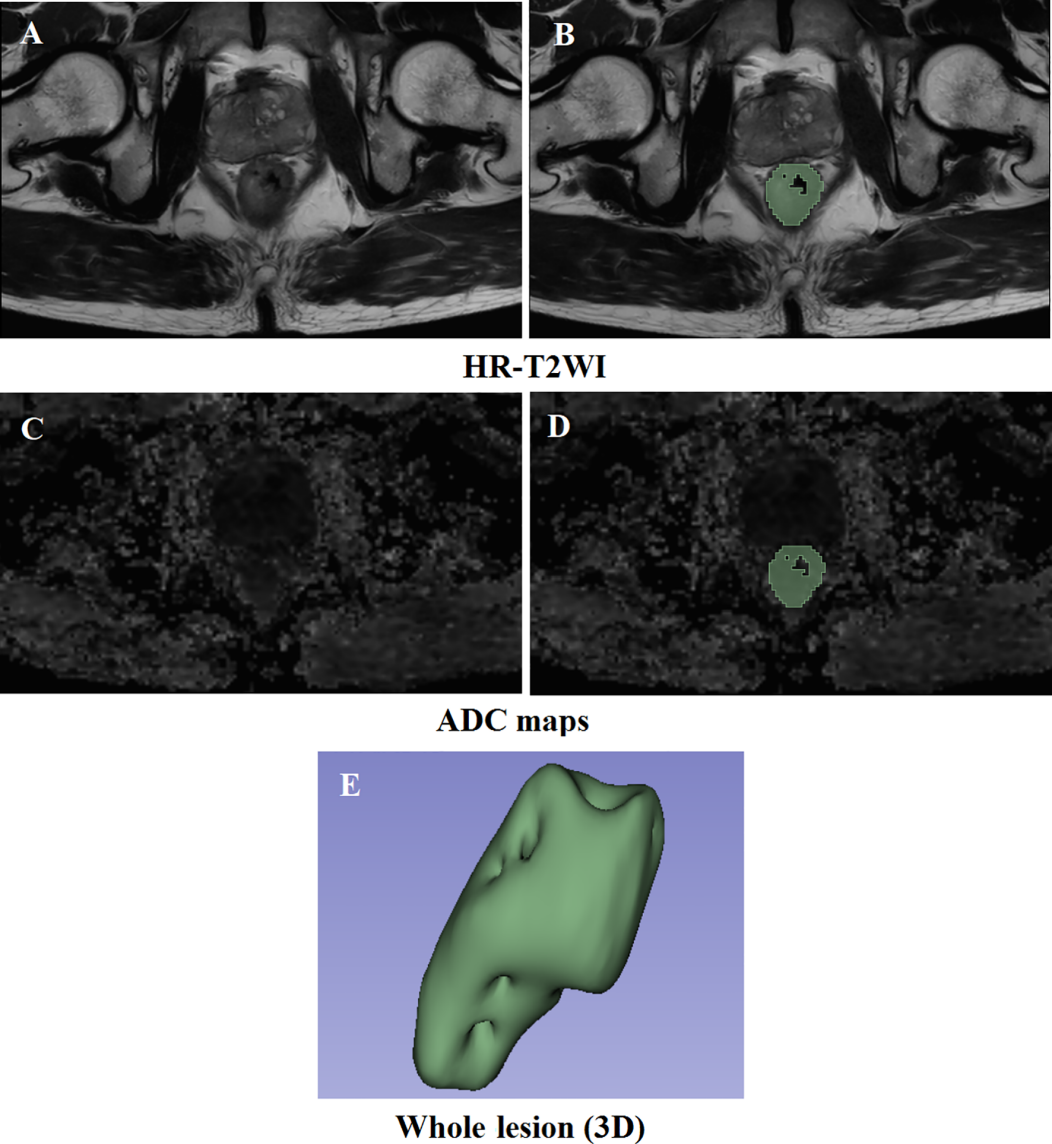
Results of the delineation of the VOI. (A, C) High-resolution T2-weighted imaging and apparent diffusion coefficient maps at the same slice level, respectively. (B, D) The result of lesion segmentation. The green marked part is the delineated lesion. (E) The three-dimensional label of the whole tumor.
